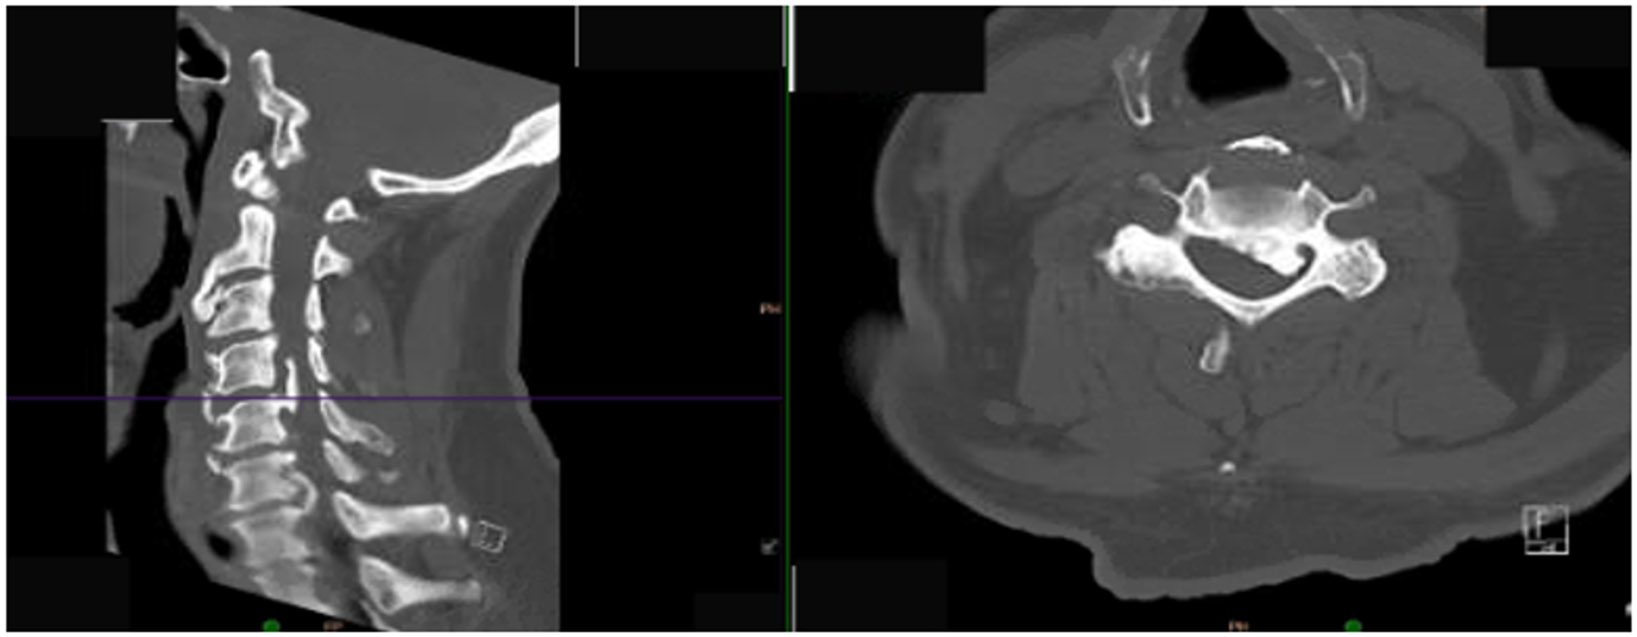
CT of cervical spine demonstrating severe stenosis with clear evidence of impingement of cord at C4-5 and C6-7 with prominent ossification of posterior longitudinal ligament and calcification.
